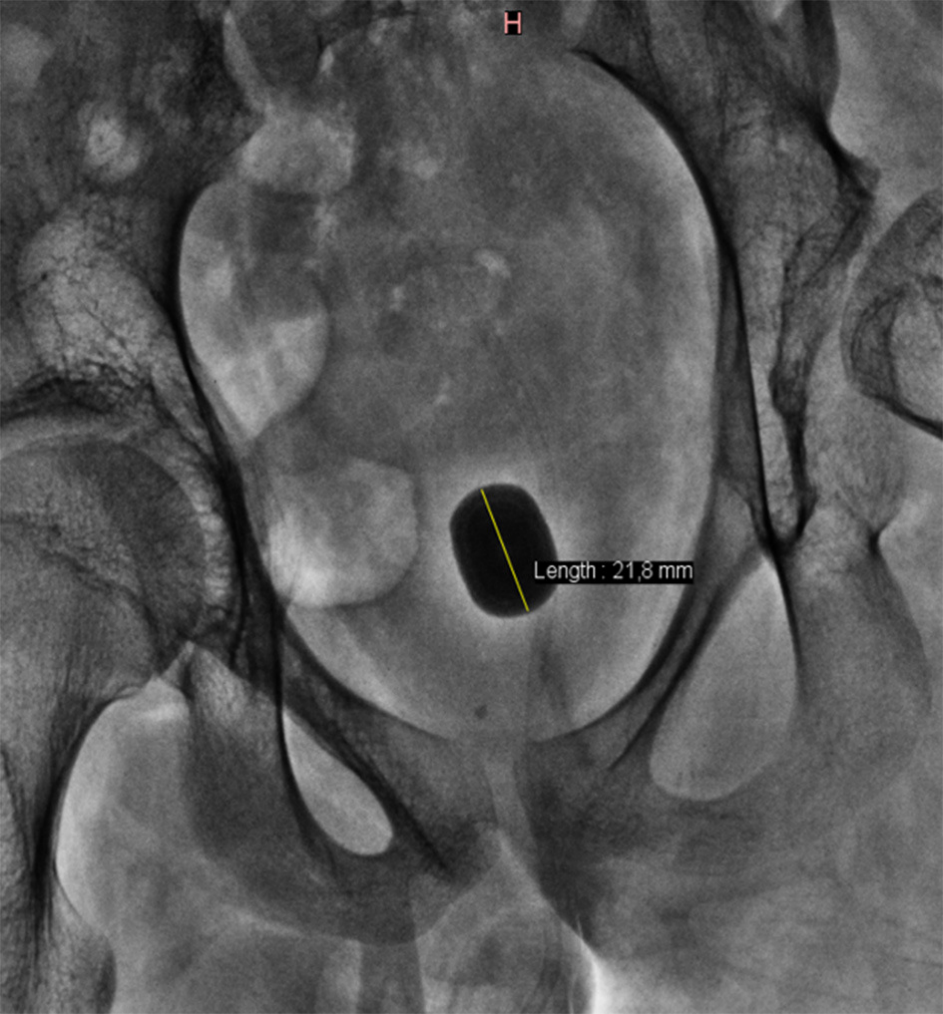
X-ray showing a big bladder stone.
